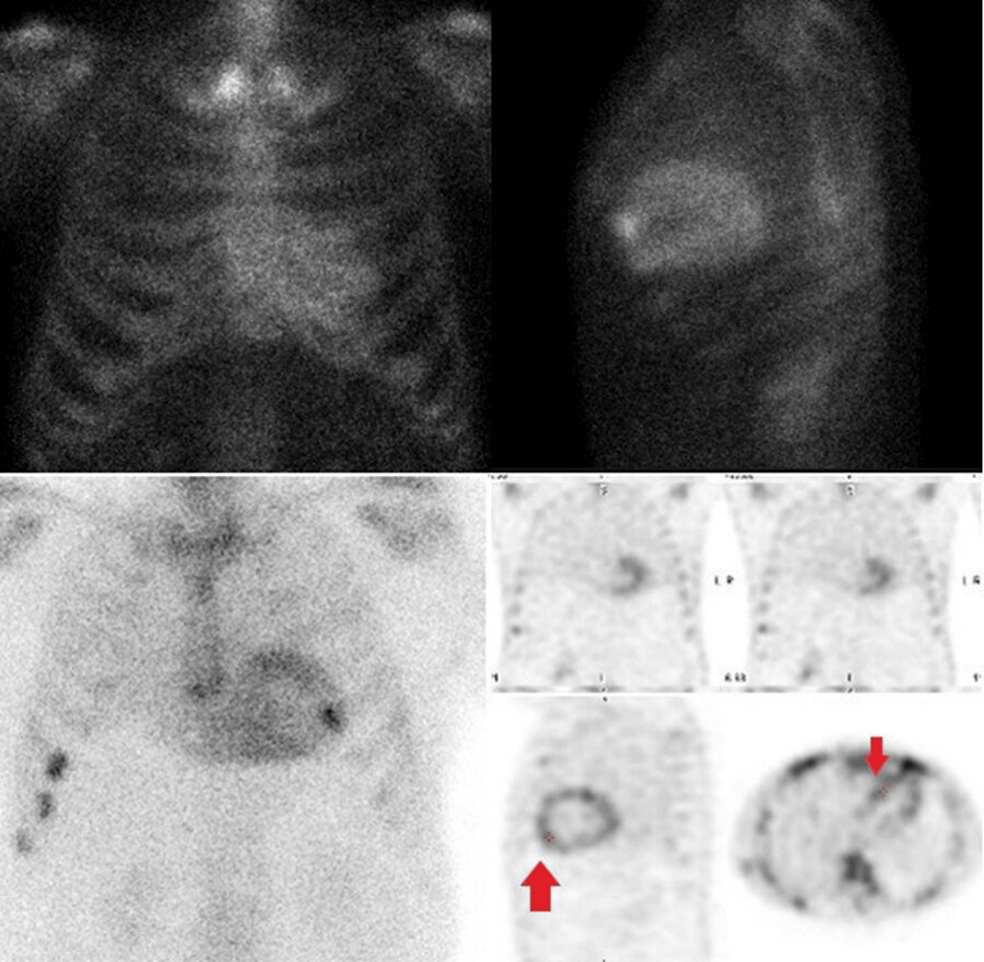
Bone scintigraphy using technetium-99m with increased cardiac uptake (Perugini score of 2) Red arrows show elevated cardiac uptake of technetium-99m.
